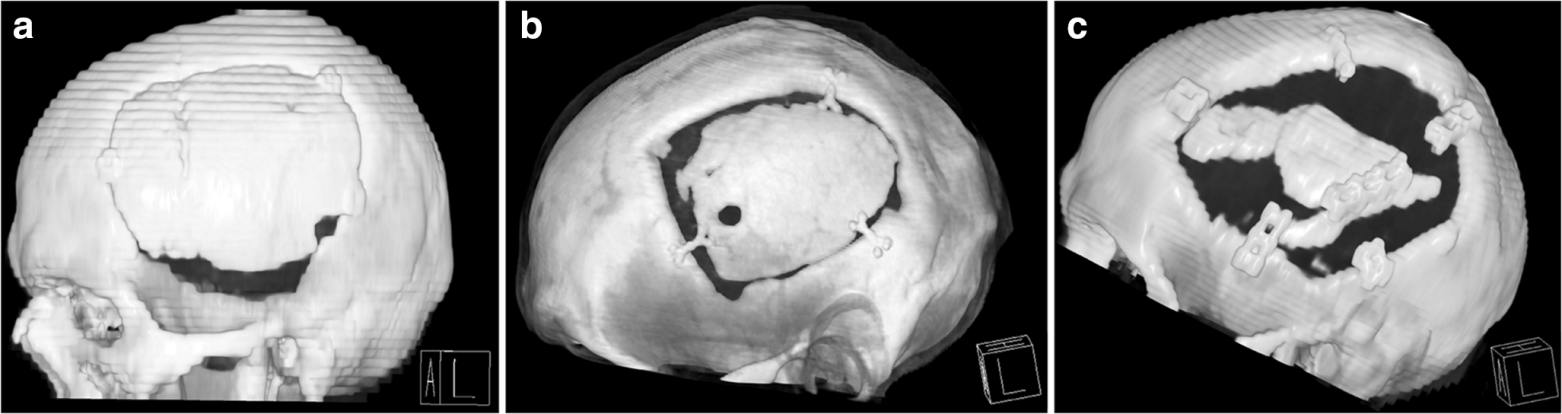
Three-dimensional cranial computed tomography reconstruction images showing examples of bone graft resorption graded as a mild: less than 50% of the graft circumference affected, b moderate: more than 50% of the graft circumference affected, and c severe: more than 50% of the original bone flap volume affected
